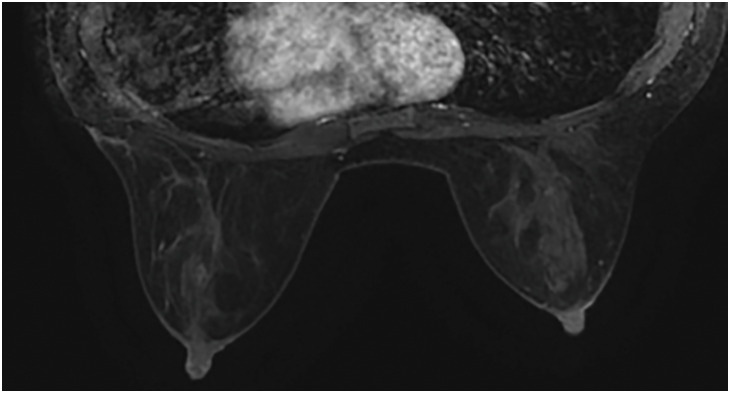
MRI 1-month post-antimicrobial therapy showing that the previous findings in the left breast had disappeared.
